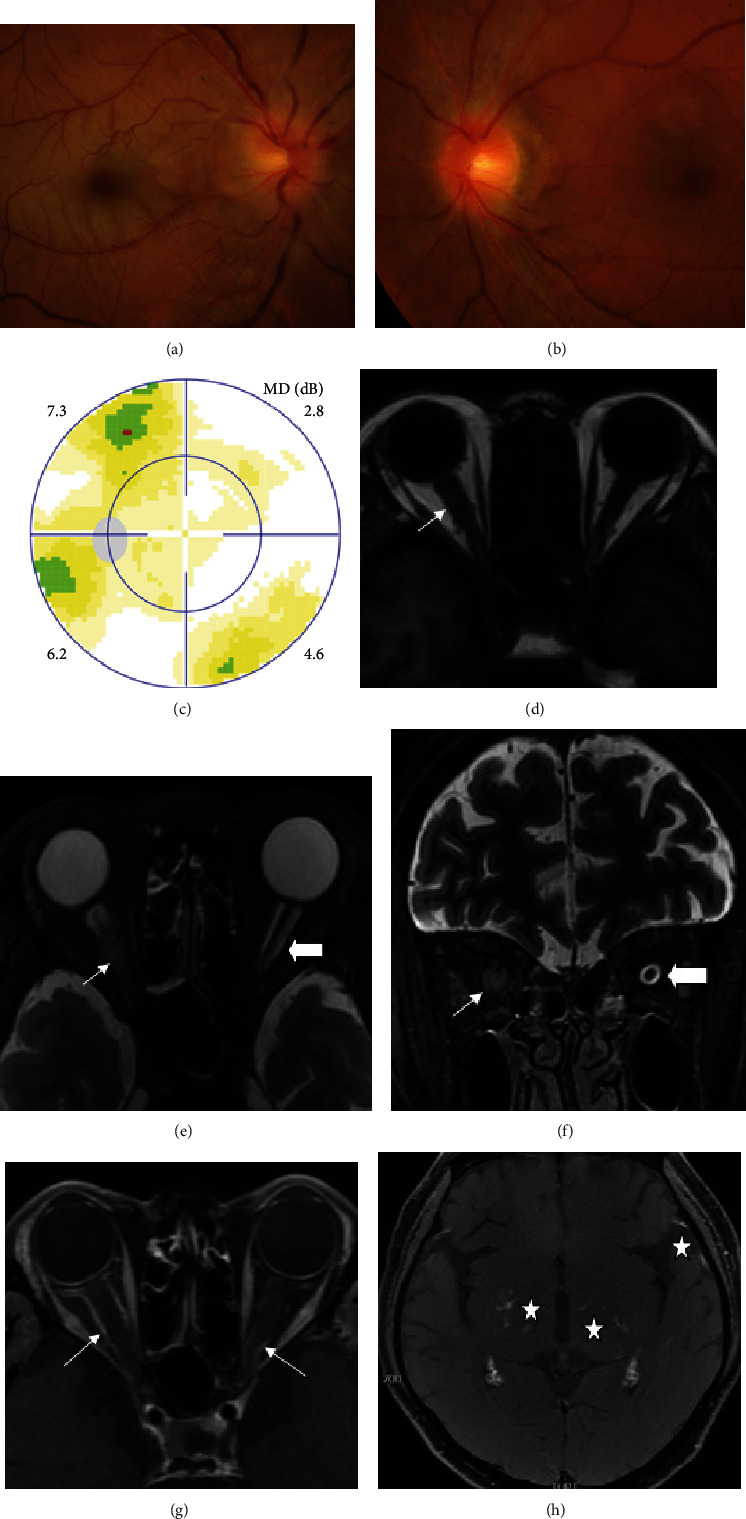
Ophthalmic and MRI examination of infiltrative optic neuropathy (right eye) and meningeal carcinomatosis-associated optic neuropathy (left eye) with leukemia in Case 13. Fundus photographs showed moderate swollen optic disc and posterior retinal edema with macular cherry red in the right eye (A), and mild swollen optic disc in the left eye (B). Visual field test was normal in the left eye (C). MRI showed enlarged optic nerve (short arrow, D, E, F) with sheath enhancement (long arrow, G) in the right eye, enlarged optic nerve sheath space (arrowhead, E, F) with sheath enhancement (long arrow, G) in the left eye. Focal linear enhancement of the meninge, and spotty and linear lesions with enhancement in basal ganglia (star, H) which supporting meningeal carcinomatosis found in T1-weighted MRI with contrast enhancement.
